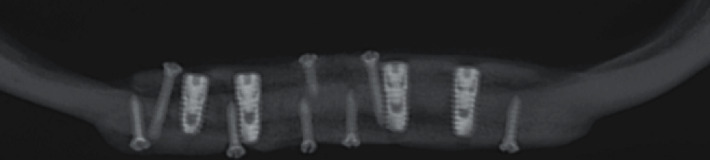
Reconstructed panoramic radiograph of patient's cone beam computed tomography (CBCT)—4-month post-operation.
